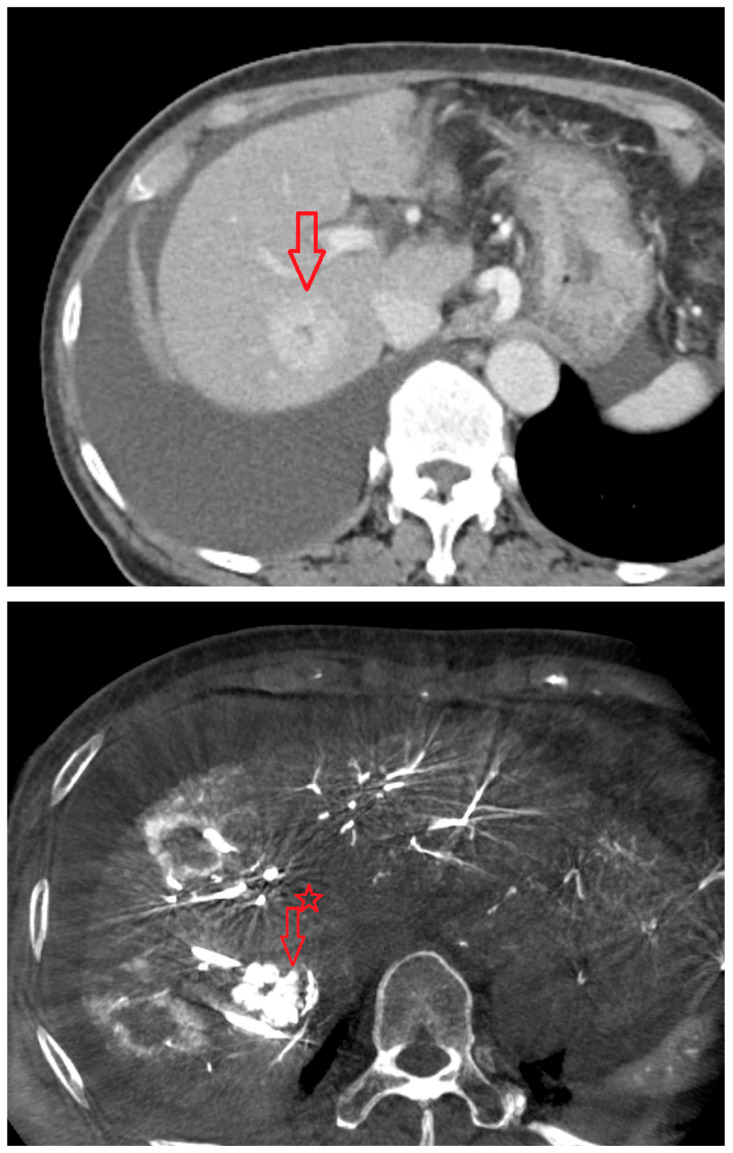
CT images from a patient with hepatocellular carcinoma undergoing angio CBCT-guided RFA. (Top): pre-procedural CT (portal venous phase; lesion 128.7 HU, red arrow = HCC mass). (Bottom): intraprocedural angio CBCT (lesion 1499.7 HU, red arrow with star = HCC mass). ΔHU = 1298.0 HU. (intra lesion − intra background) − (pre lesion − pre background).
